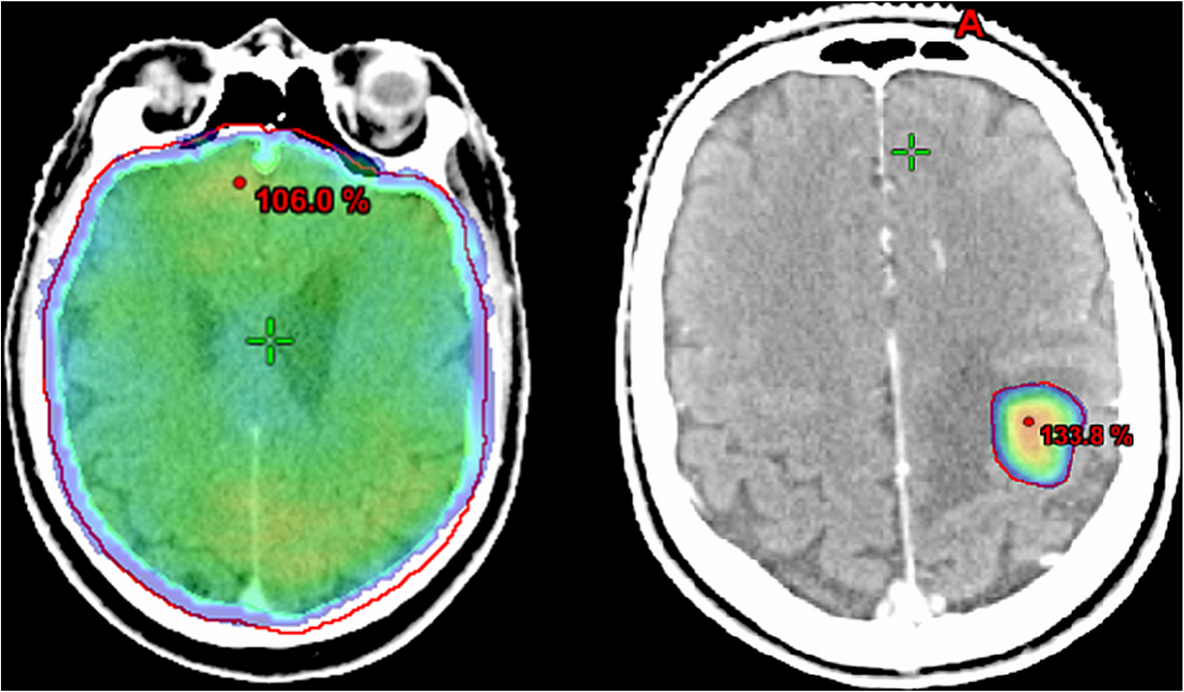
Dose distribution difference between WBRT (*left*) and SRS (*right*). A typical dose distribution on a planning-CT of WBRT on the left side and SRS on the right side. With WBRT, the healthy brain tissue receives the same low palliative radiation dose (non-ablative). With SRS, only the metastatic tissue receives a high ablative dose
